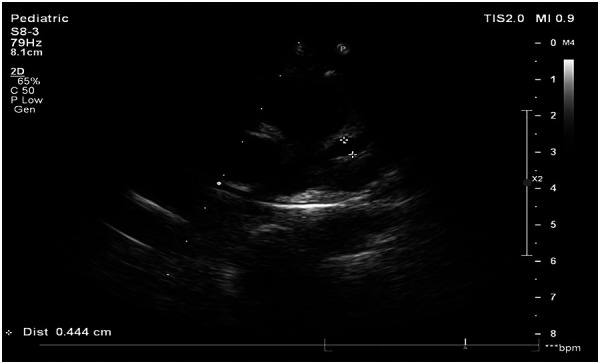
Echocardiography of the left anterior descending coronary artery on the 11th day of hospitalization (*Z*-score 8.46). *Z*-scores calculated as per Figure 2.
